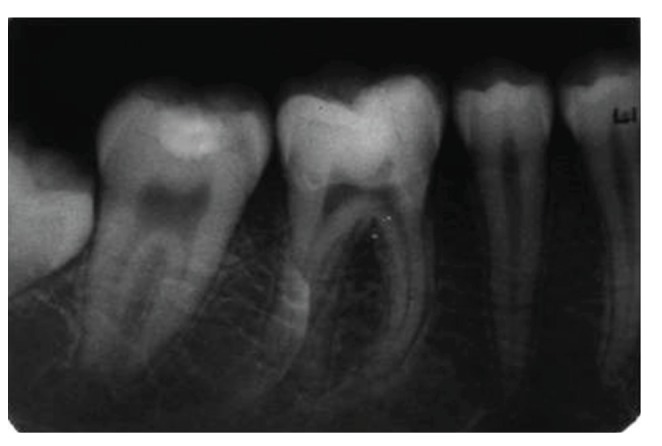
Intraoral periapical radiograph with 46 at 2 years followup. No significant pulpal or periapical changes.
